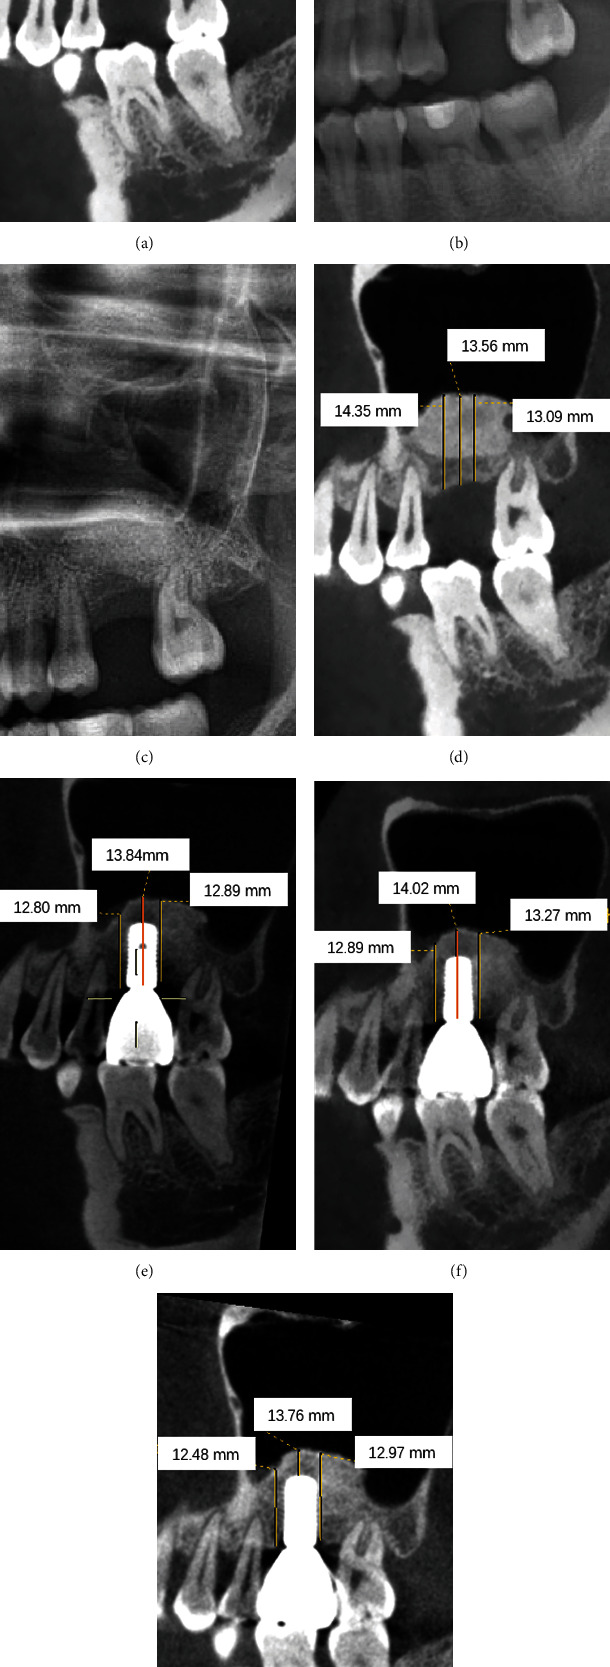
CBCT and pantomograph follow-up for tooth #26. (a) Before MSFE. (b) Immediately after MSFE (pantomograph). (c) 3 months after MSFE (pantomograph). (d) 7 months after MSFE. (e) 14 months after MSFE. (f) 20 months after MSFE. (g) 32 months after MSFE.
